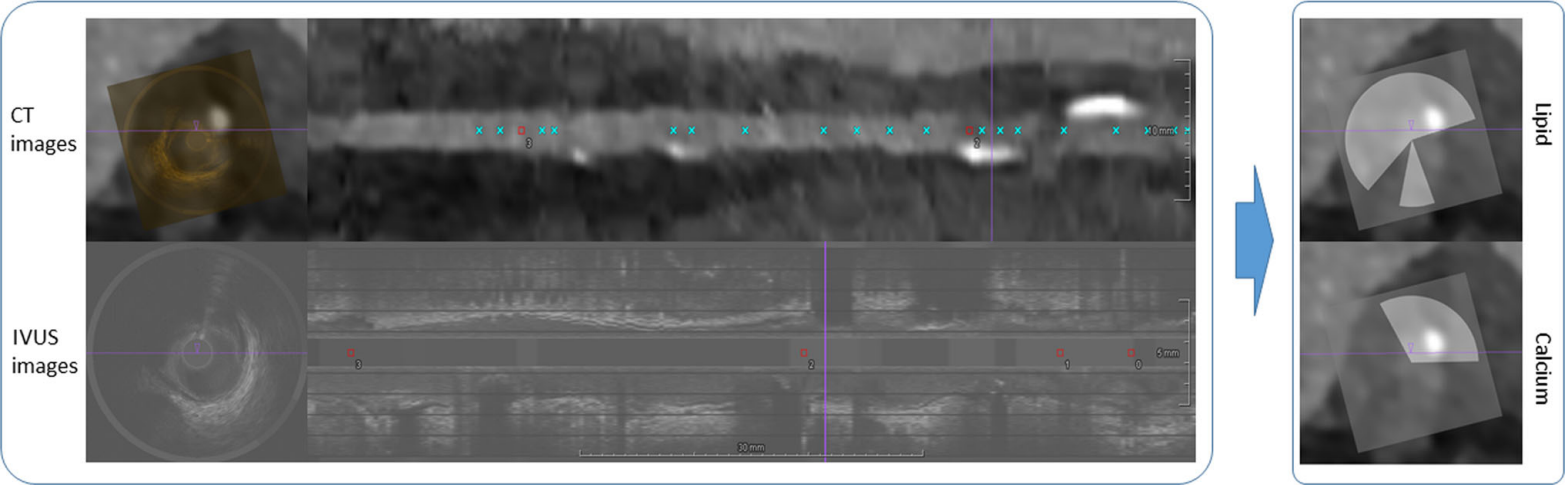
Vessel registration process to map IVUS images onto CT images
